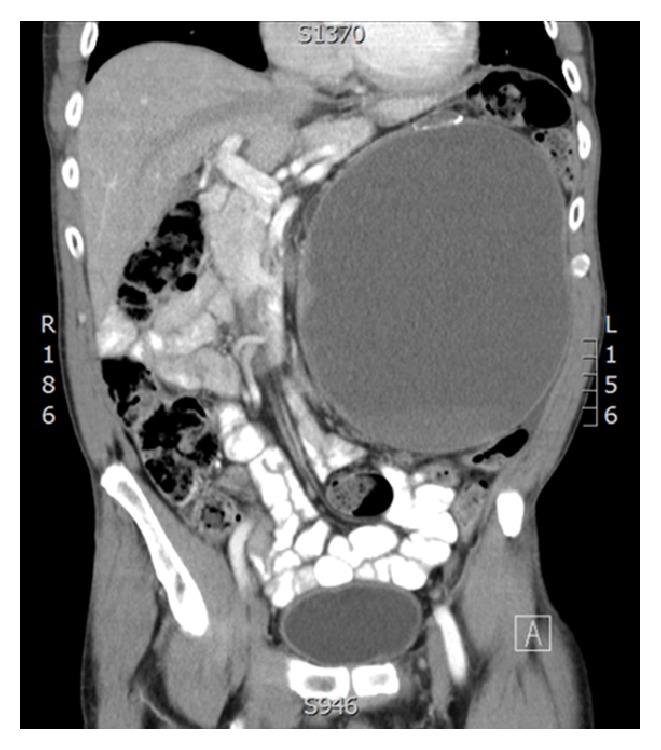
CT abdomen coronal view showing a large left cystic renal mass displacing great vessels laterally.
